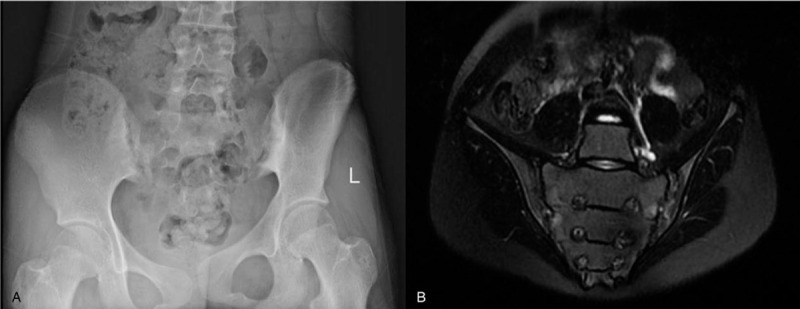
Case 1. (A) x-Ray showing a blurred surface of the sacroiliac joints bilaterally, with elevated density on the iliac side. (B) Magnetic resonance imaging showing coarse sacroiliac joint surfaces with disseminated patches of long T1 signals. The changes are consistent with ankylosing spondylitis.
